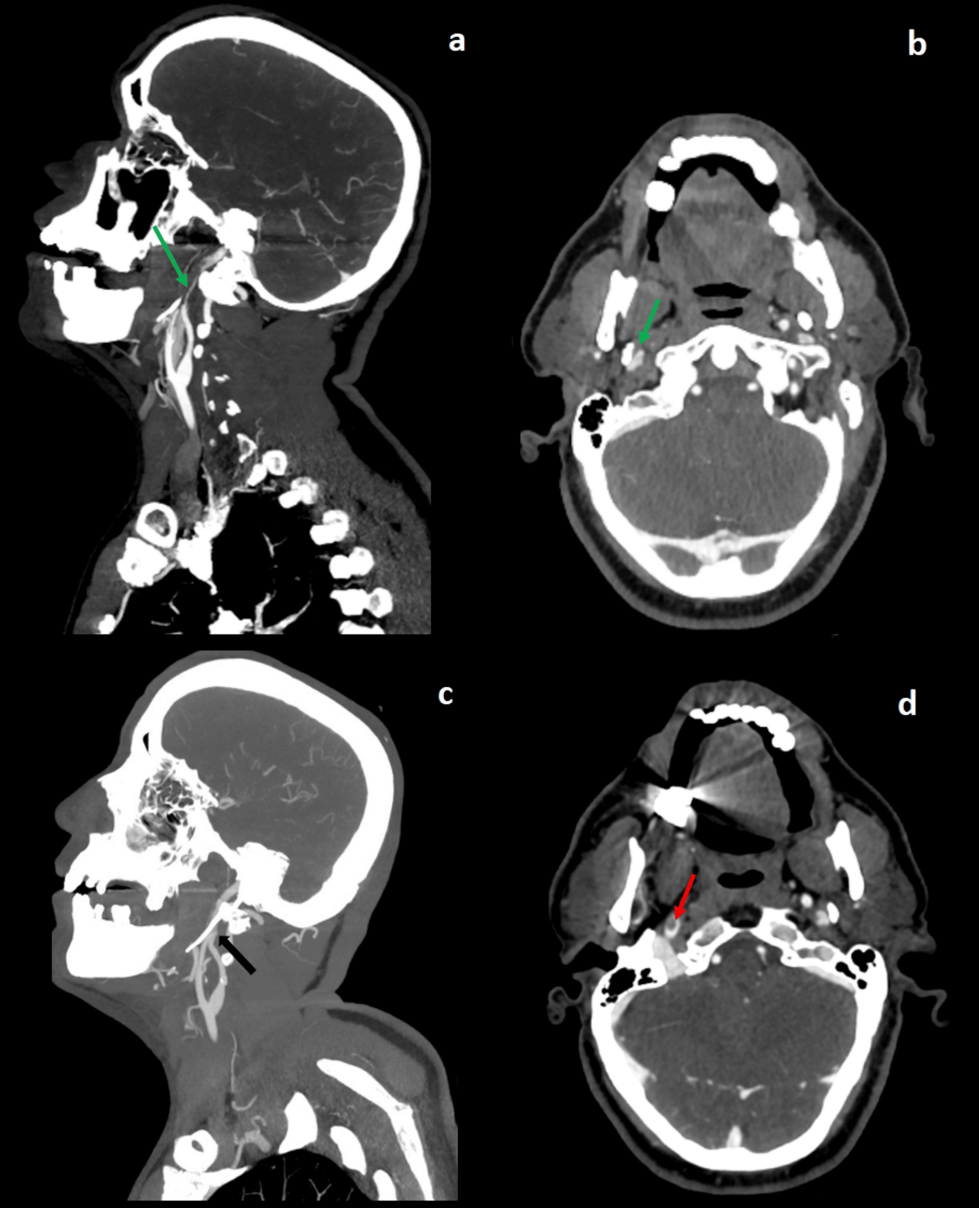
Case 2 sagittal (a, c) and axial (b, d) CT images showing an ICA dissection (green arrows) due to mechanical conflict with an elongated styloid process (c) (black arrow) complicated by a floating thrombus (d) (red arrow) CT: computed tomography, ICA: internal carotid artery
